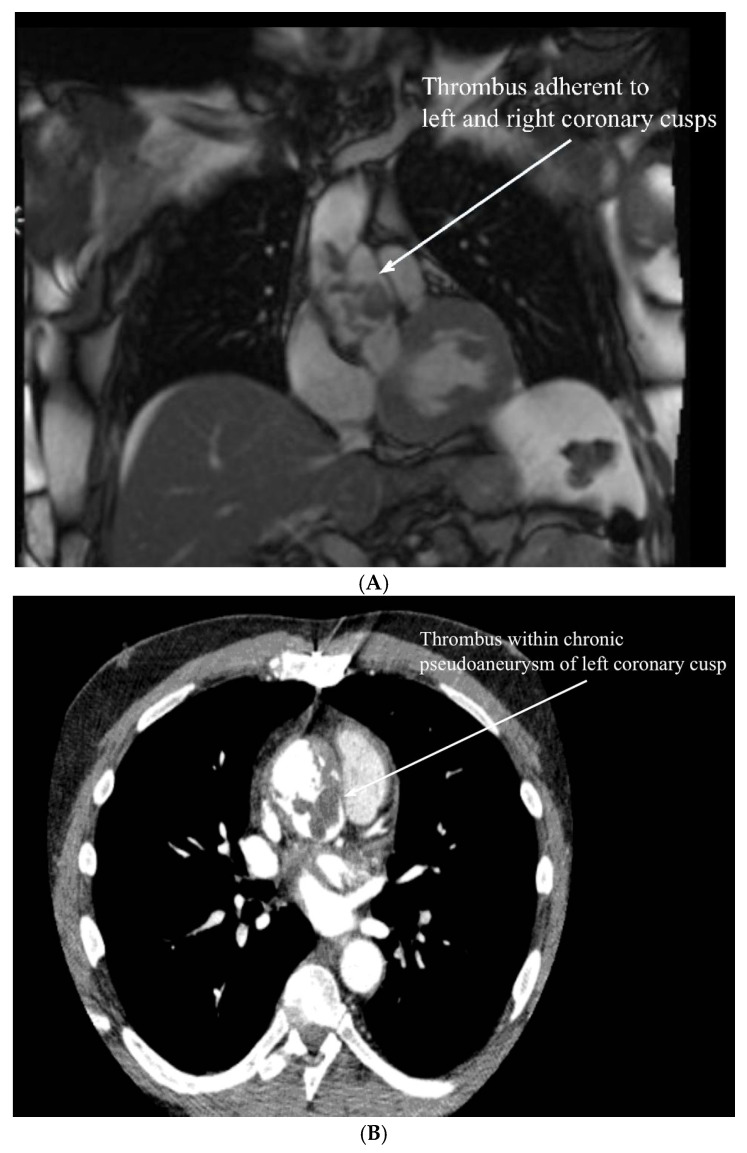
(A). MRI showing a thrombus extending from the aortic valve leaflets up to the level of the distal anastomosis of the interposition aortic graft. (B). CT scan showing thrombus within pseudoaneurysm of left coronary cusp.
